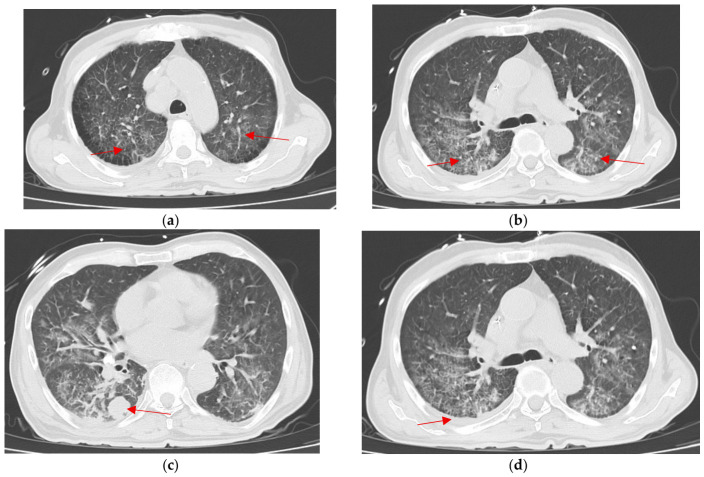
(a,b) Chest computed tomography demonstrates alveolar consolidation with a peribronchovascular distribution, predominantly involving the dependent regions and affecting both lung parenchyma. These findings are associated with multilobar areas of ground glass opacities, defined as regions of increased lung attenuation with preservation of underlying bronchovascular structures (arrows) (c) Additionally, a solid nodular lesion measuring approximately 23 × 20 mm is identified in the posterior segment of the lower right lobe, (d) A small right-sided pleural effusion is present, along with subsegmental atelectasis in both lower lobes.
